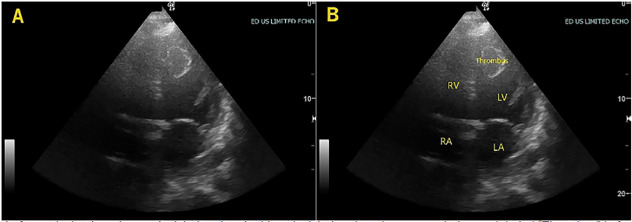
Left ventricular thrombus, apical 4-chamber. In this apical 4-chamber view, a rounded mass labeled “Thrombus” in frame B is seen in the apex of the left ventricle (LV). *RV*, right ventricle; *RA*, right atrium; *LA*, left atrium.
